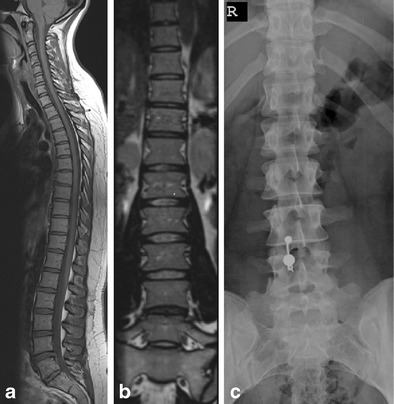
26-year-old woman with thoracic pain. MRI of the spine with sagittal T1-weighted images (a) and a coronal reformat of a sagittal SPACE sequence (b) demonstrates a transitional lumbosacral vertebra. Counting from the top, this is a sacralised L5 vertebra. The coronal reformats are very helpful in identifying the transitional nature of this vertebra. It is more easily appreciated on an anterior-posterior (AP) radiograph of the lumbar spine (c)
